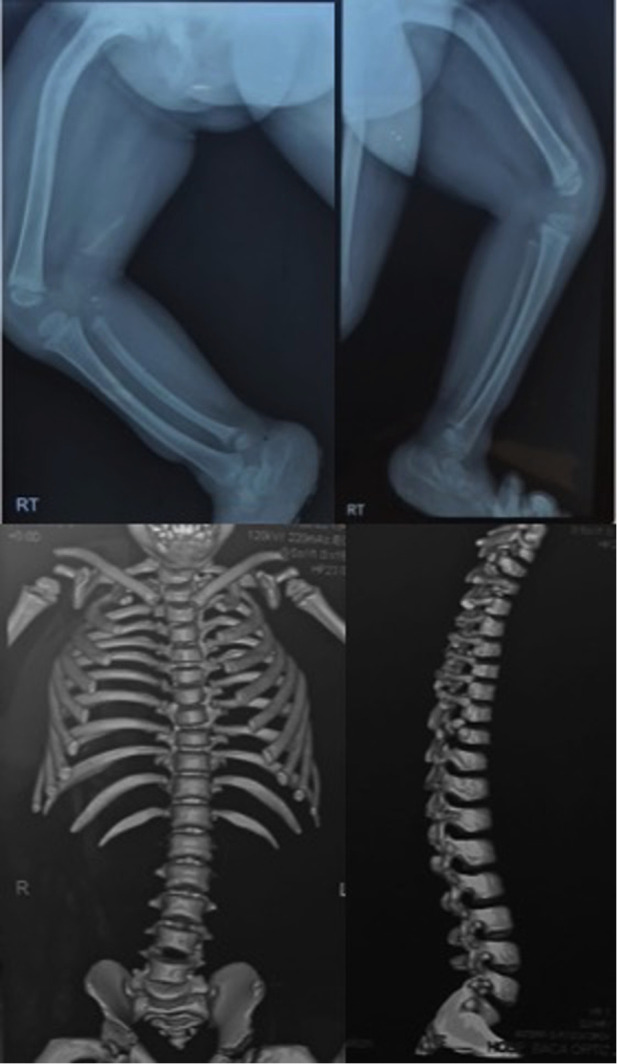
Radiography and computed tomography of the legs, hip, and spine showing femoral, tibial, and fibular bowing, hip rotation, and an abnormal spine curvature.
